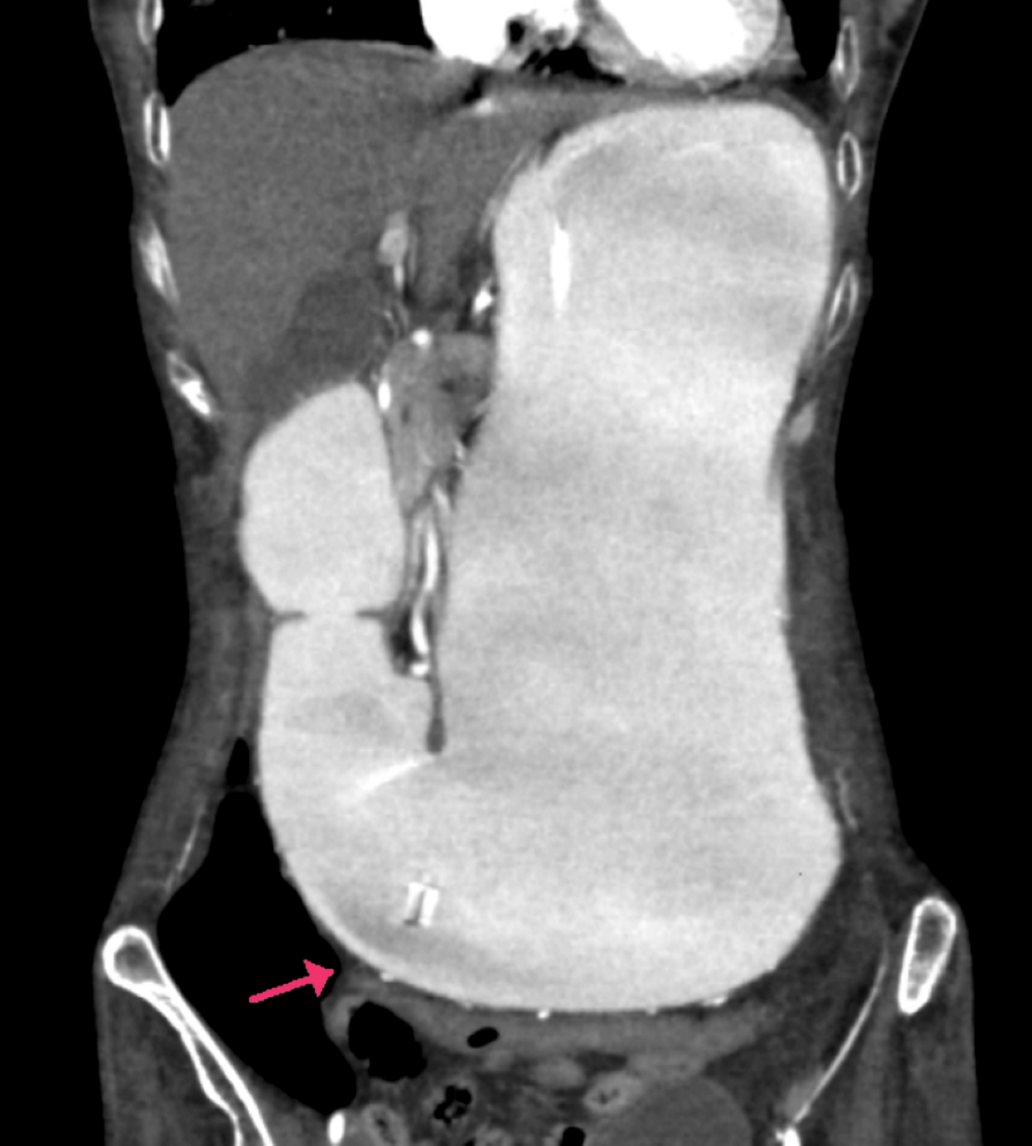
Contrast-enhanced computed tomography (CECT) of the abdomen revealed overdistention of the stomach with greater gastric curvature reaching toward the pelvic inlet
